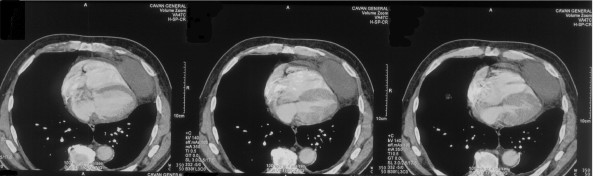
CT Scan of the thorax showing the pericardial cyst.
